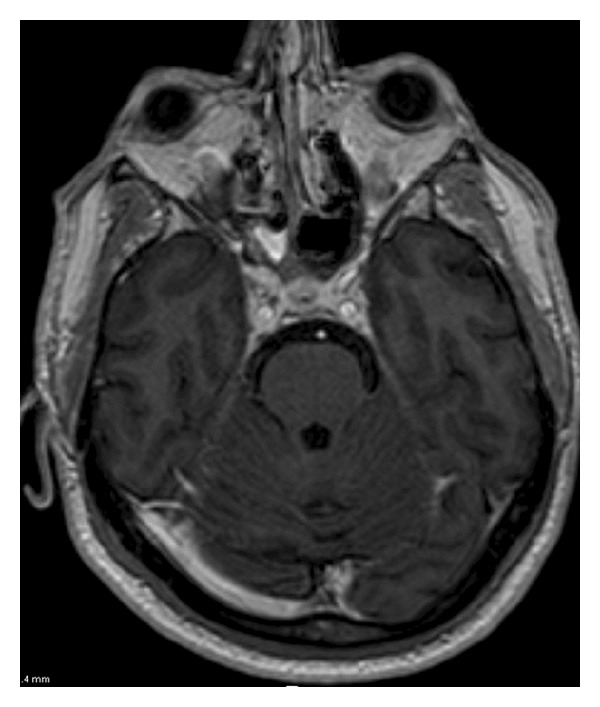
Axial T1-weighted MRI with gadolinium contrast showing a subtotal response 6 months after-radiotherapy.
